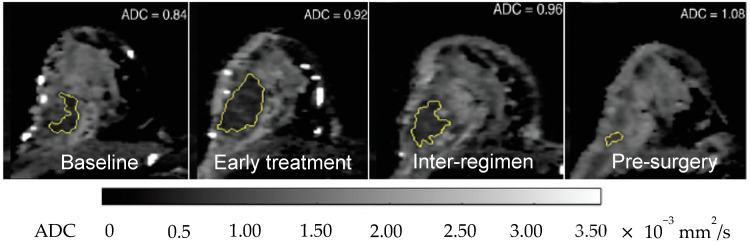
An example of an HR+/HER2− breast cancer patient who had neoadjuvant chemotherapy being monitored by MRI. The serial apparent diffusion coefficient (ADC) maps, obtained from diffusion-weighted (DW) MRI (b = 800 mm^2^/s), show an increase in value with treatment. Compared to baseline, ∆ADC = +9.5% at early treatment; +14% at inter-regimen and +29% at pre-surgery.
